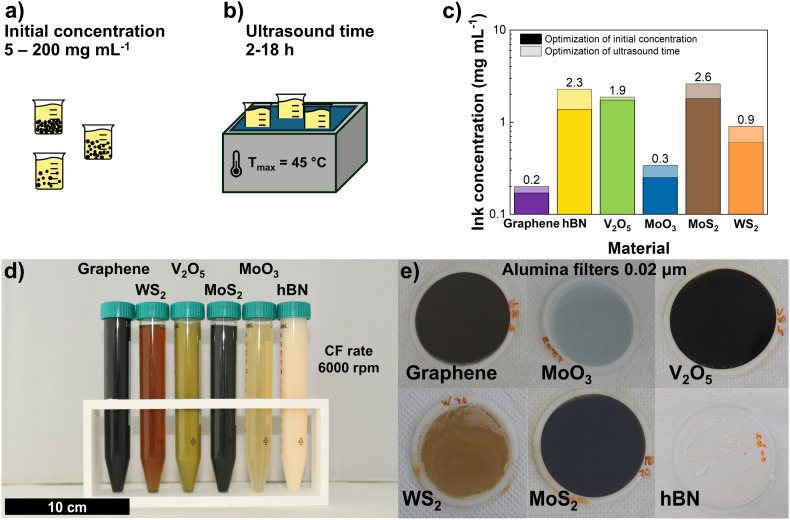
Optimization of final concentration by varying: (a) initial concentration (5–200 mg mL^−1^) and (b) ultrasound time (2–18 h). (c) Highest concentrations obtained in each series for all materials. (d) Optimized inks and (e) filtered inks in 0.02 μm alumina filters.
